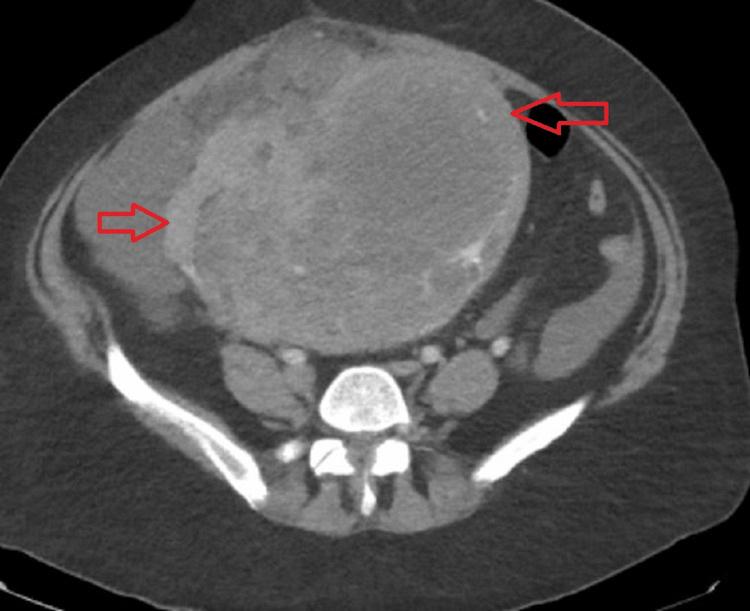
CT abdomen and pelvis with IV contrast demonstrating an enlarging uterus with a heterogeneous mass (red arrows). Specifically, the uterus has increased by 10 cm in 2 months CT: computed tomography
